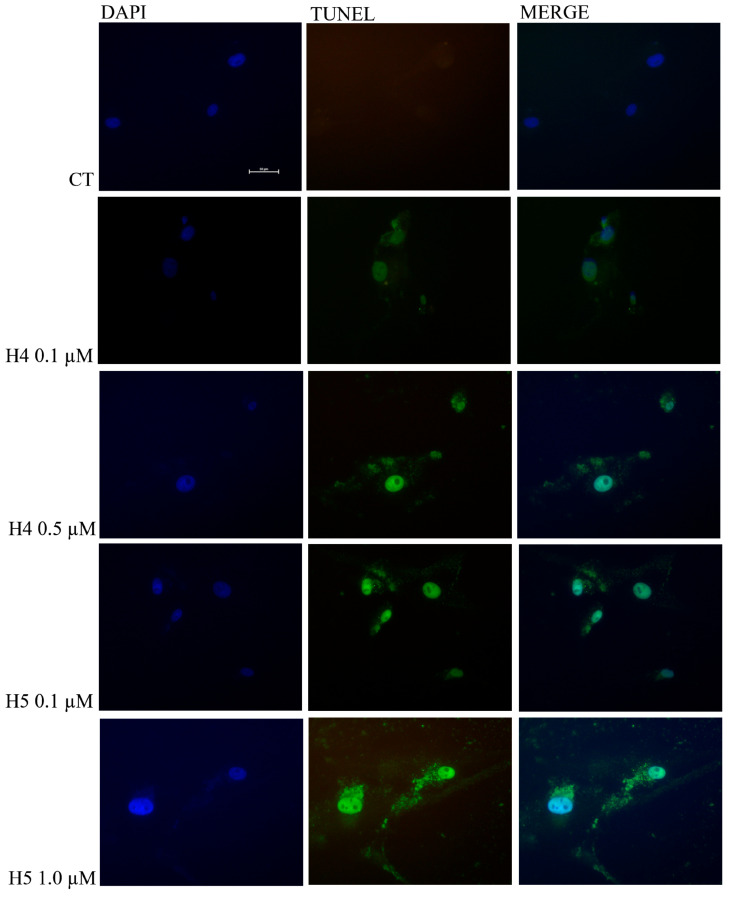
Representative images from three independent experiments (n = 3) after TUNEL assay of U87MG treated with hortensin 4 (H4; 0.1 and 0.5 μM) and hortensin 5 (H5; 0.1 and 1.0 μM) for 72 h compared with untreated U87MG (CT). Magnification 40×. Scale bar, 50 µm.
